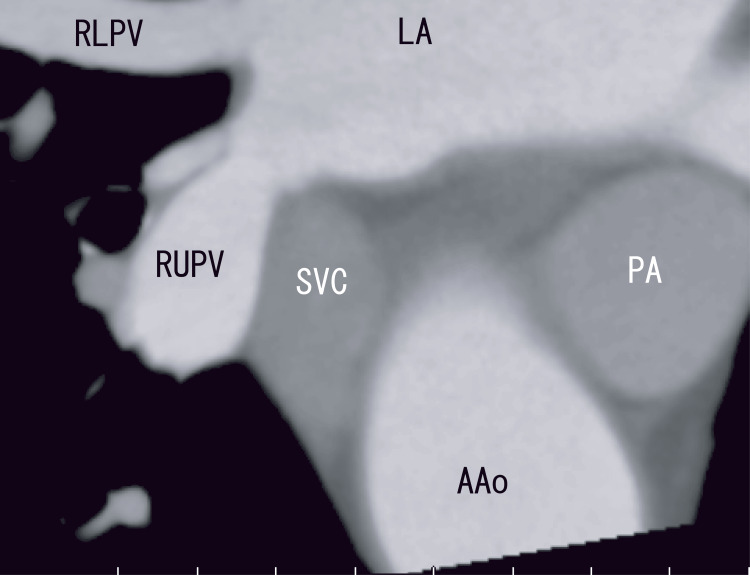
Oblique enhanced CT images showing the AAo and LA Oblique enhanced CT images clearly revealed no linear white thrombi and no changes in the AAo wall. The angle of these images is similar to that of the TEE images. AAo: Ascending aorta; LA: Left atrium; PA: Pulmonary artery; RLPV: Right lower pulmonary vein; RUPV: Right upper pulmonary vein; SVC: Superior vena cava.
